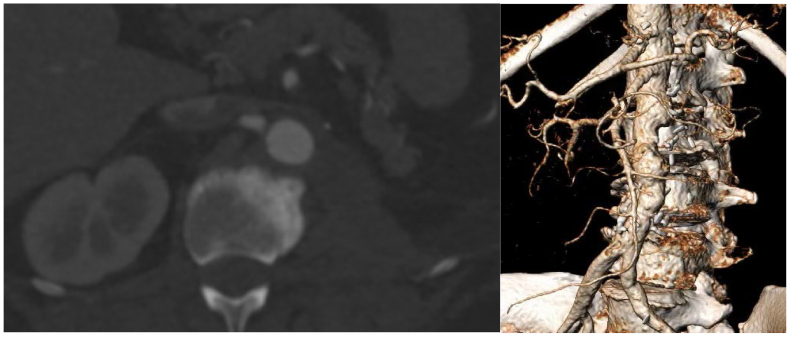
Six-week postoperative computed tomography angiography with three-dimensional reconstruction demonstrates resolution of the pseudoaneurysm following patch repair and left nephrectomy.
